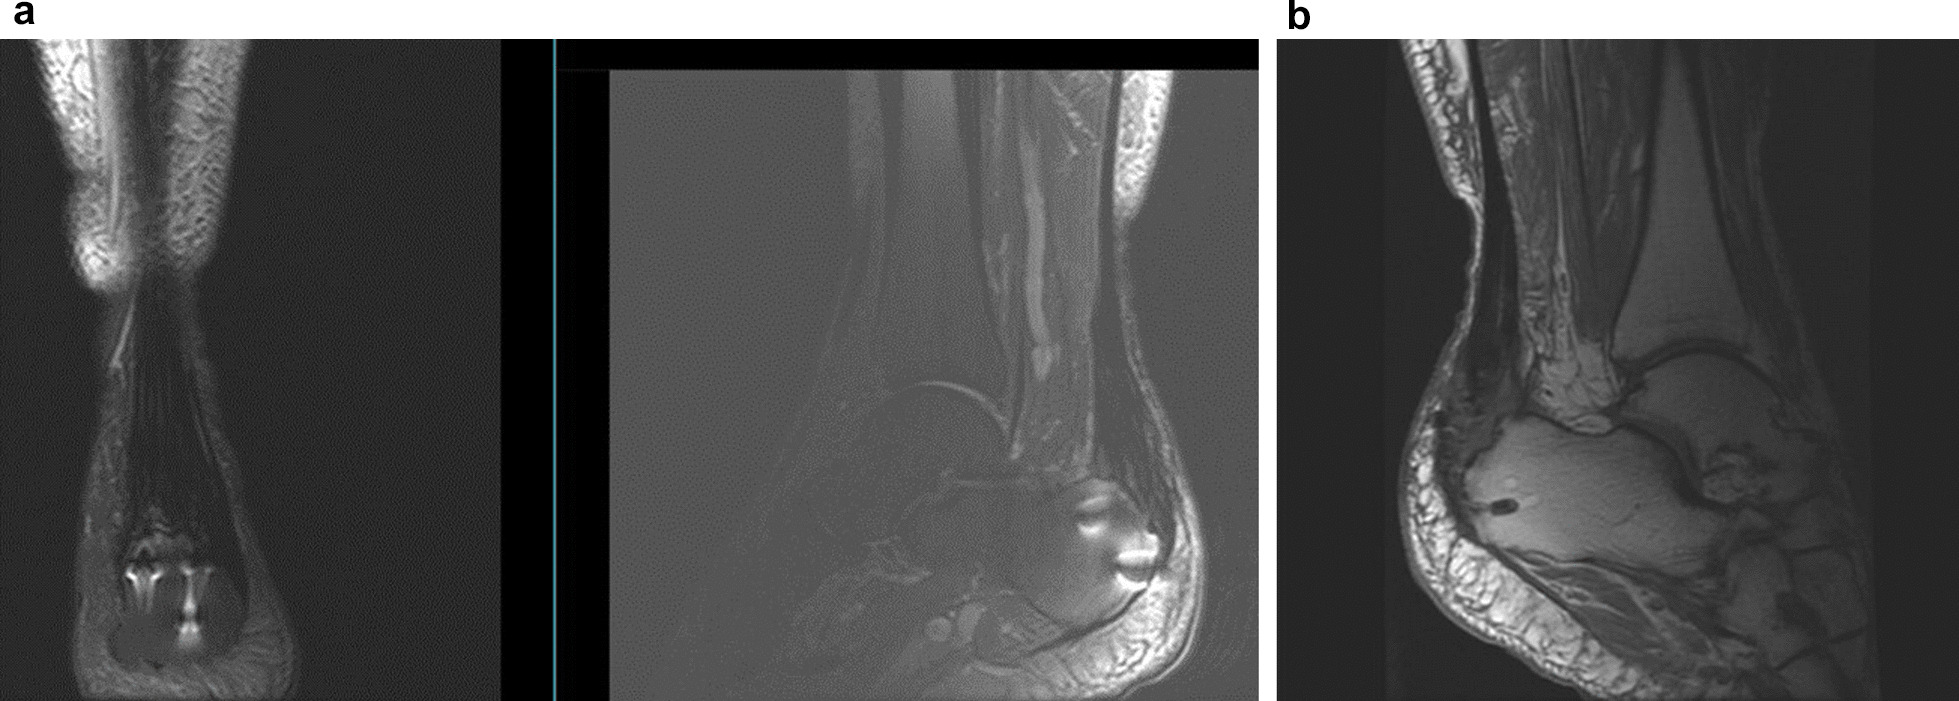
a Magnetic resonance imaging from the right ankle 12 months postoperatively. b Magnetic resonance imaging from the left ankle 12 months postoperatively
